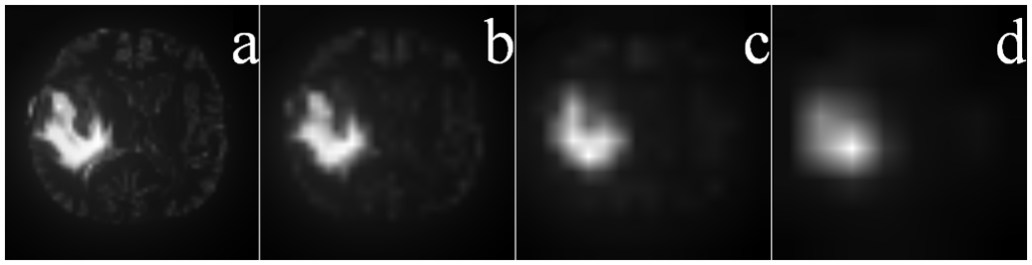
Saliency map of pseudo-colored MR image of the whole tumor region of Fig 4(a) at different scales. Patch sizes of (a) 4 × 4, (b) 8 × 8, (c) 16 × 16, and (d) 32 × 32.
